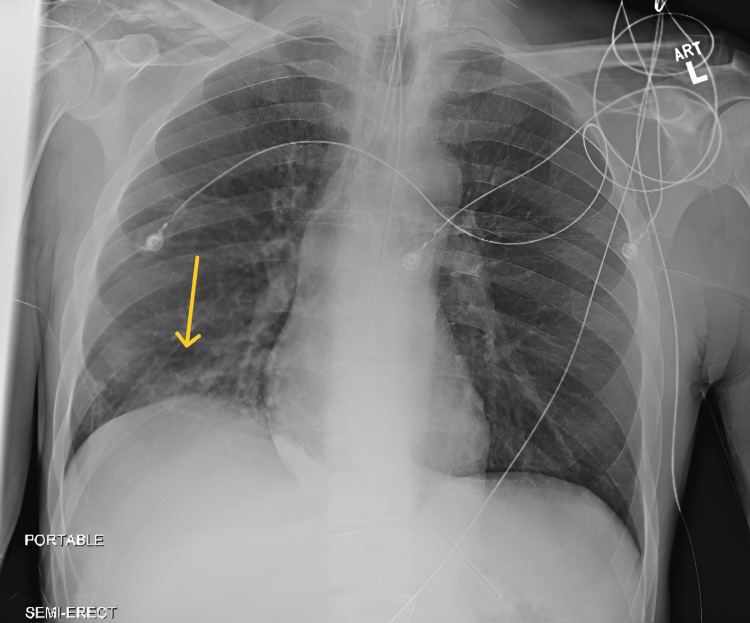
Chest radiography. The yellow arrow indicates basilar opacity.
